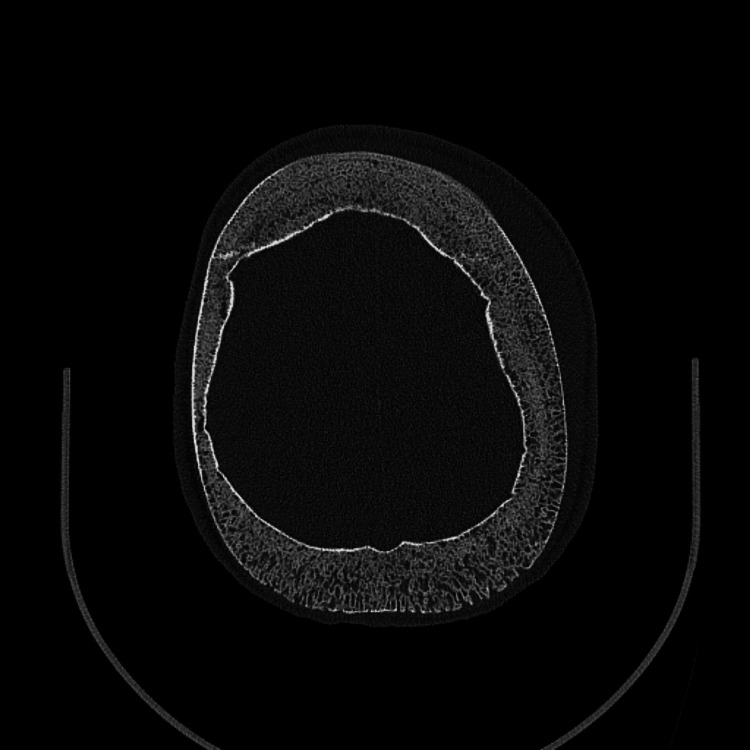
Hair-on-end appearance on head and maxillofacial CT
